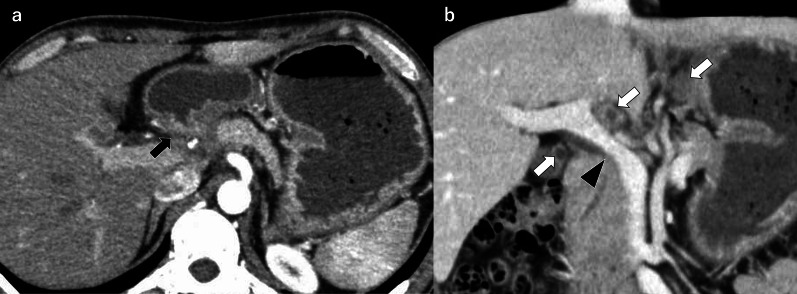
Abdominal computed tomography (CT) images on admission. a Duodenal stricture and wall thickening (black arrow). b Numerous enlarged abdominal lymph nodes (white arrows). Some of them have low CT values, suggesting necrosis. The portal vein is constricted by a low-density area along the hepatoduodenal ligament (head arrow)
